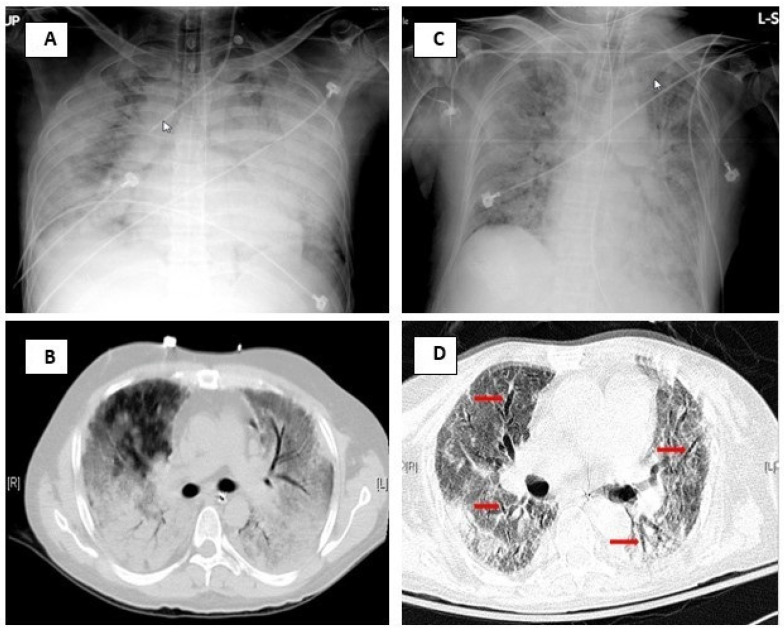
(A,B) Chest radiography and HRCT scan at the level below carina of a 35-year-old male patient with psoriasis with ARDS caused by methotrexate-induced pneumonitis who survived and was discharged. (C,D) Chest radiography and HRCT scan at the level below the carina of a 75-year-old female with left parotid gland lymphoepithelial carcinoma with liver and bone metastasis and ARDS caused by pneumonia who expired in ICU. Both chest X-rays (A,C) show bilateral dense consolidation, more severe in (A) the survival case. (B) HRCT findings of the survival case show extensive GGO and consolidation with a smooth bronchial wall. (D) HRCT findings in the non-survival case show bilateral GGO, reticulation, and prominent traction bronchiectasis (red arrows).
